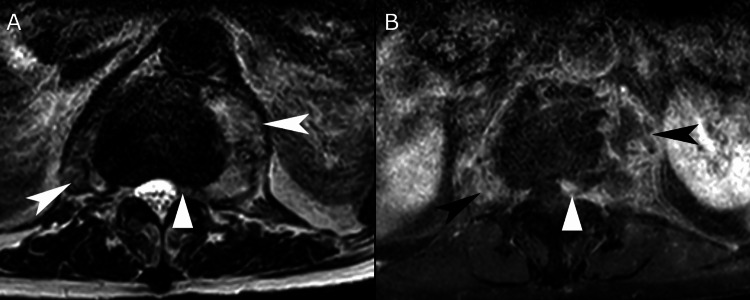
T1-weighted (A) and T1-weighted post-contrast (B) axial lumbar magnetic resonance imaging at the level of the T12/L1 intervertebral disc A rim contrast-enhancing psoas abscess with a thick and irregular wall is evident bilaterally, larger on the left (arrowheads), which extends into the epidural space (triangle).
